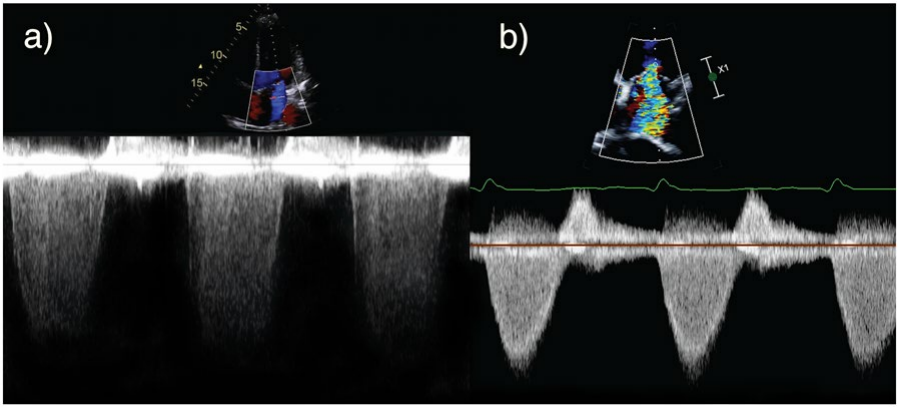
Continuous wave Doppler. Incomplete, parabolic Doppler signal in mild MR (a) versus dense, triangular shaped Doppler signal in severe MR (b)
